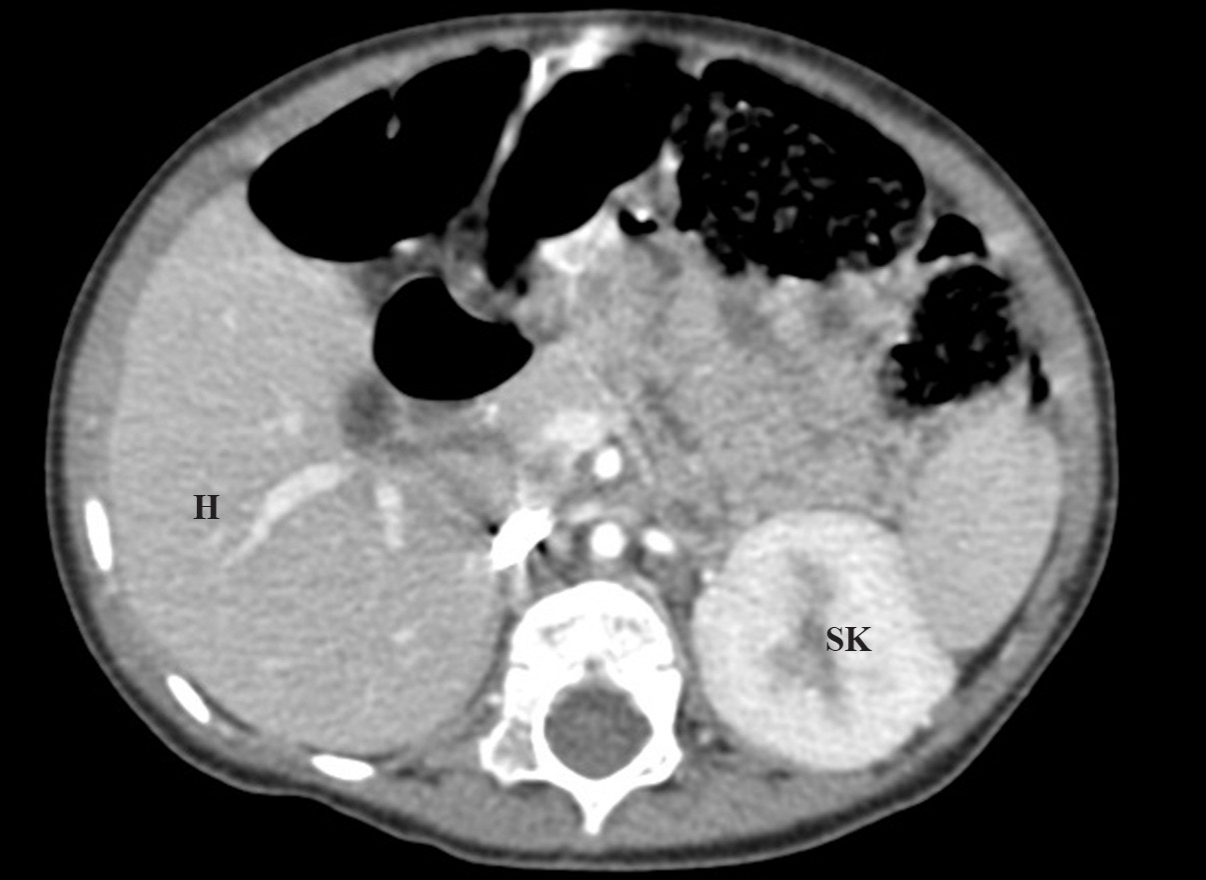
Abdominal computed tomography scan, axial view, showing apparent hepatomegaly and a single kidney.
